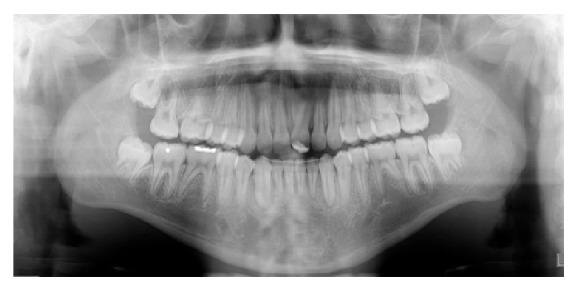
Stage 1: Orthodontic Treatment Final Panoramic Radiograph.
